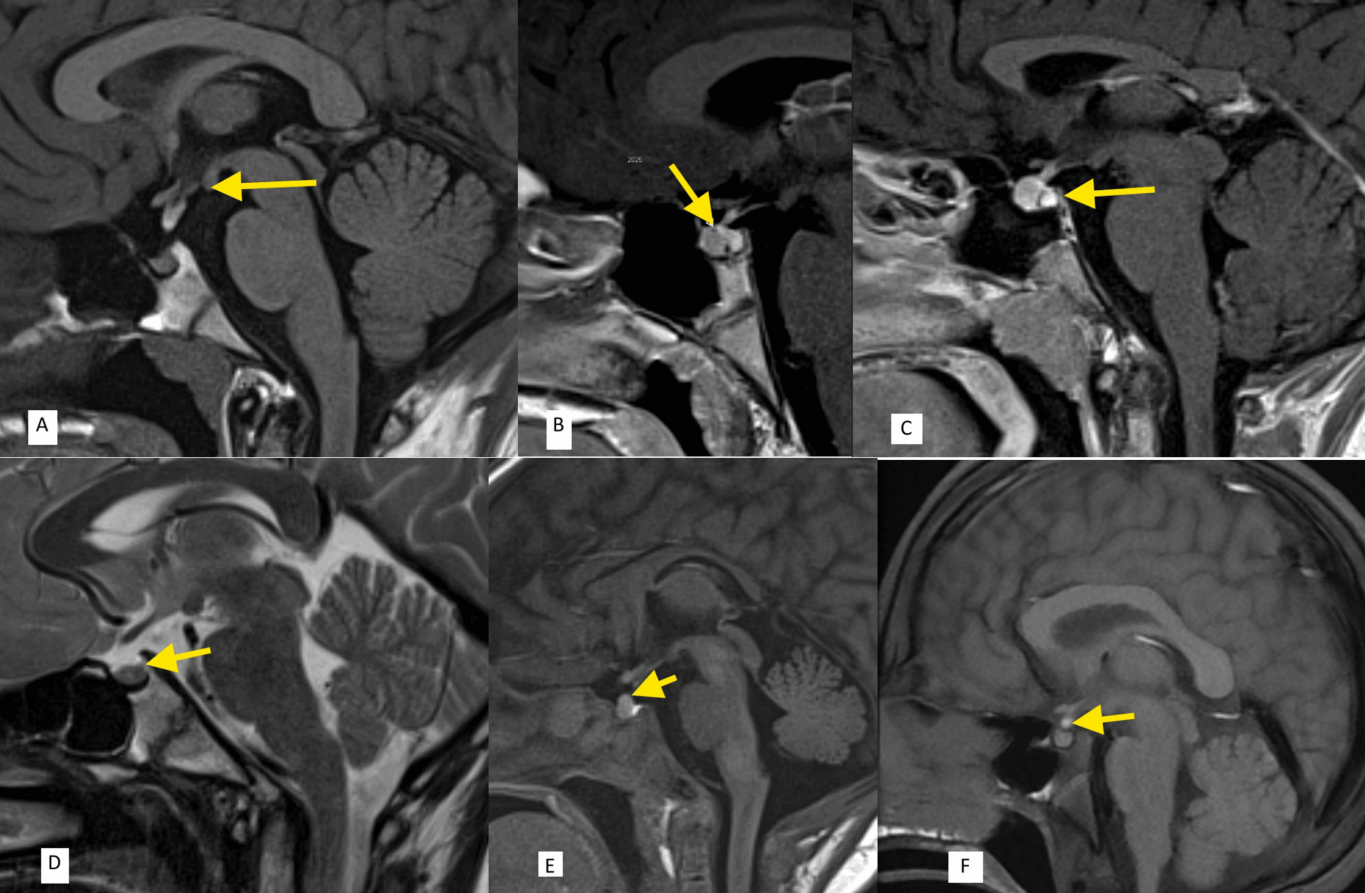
MRI images of pituitary lesions: (A) interrupted stalk syndrome (arrow); (B) microadenoma (arrow); (C) pituitary bulge (arrow); (D) Rathke's cleft cyst (arrow); (E) pituitary apoplexy (arrow); (F) ectopic posterior pituitary (arrow).
